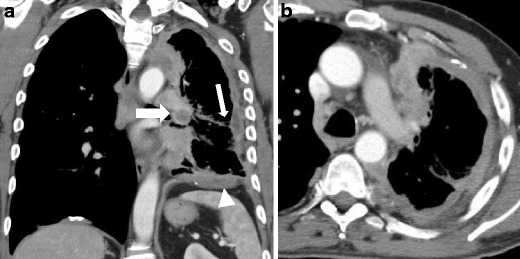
Stage III of MM. Contrast-enhanced CT scan: coronal (a) and axial (b) view show a severe left lung hypoexpansion, with circumferential irregular pleural thickening and fissure thickening (*white arrow*); left hilar inhomogeneous pathological lymph node may be seen (*thick arrow*), and infiltration of the left hemidiaphragm is reported (*arrowhead*); this MM (sarcomatoid mesothelioma variant) is classified as T2, N1, M0
